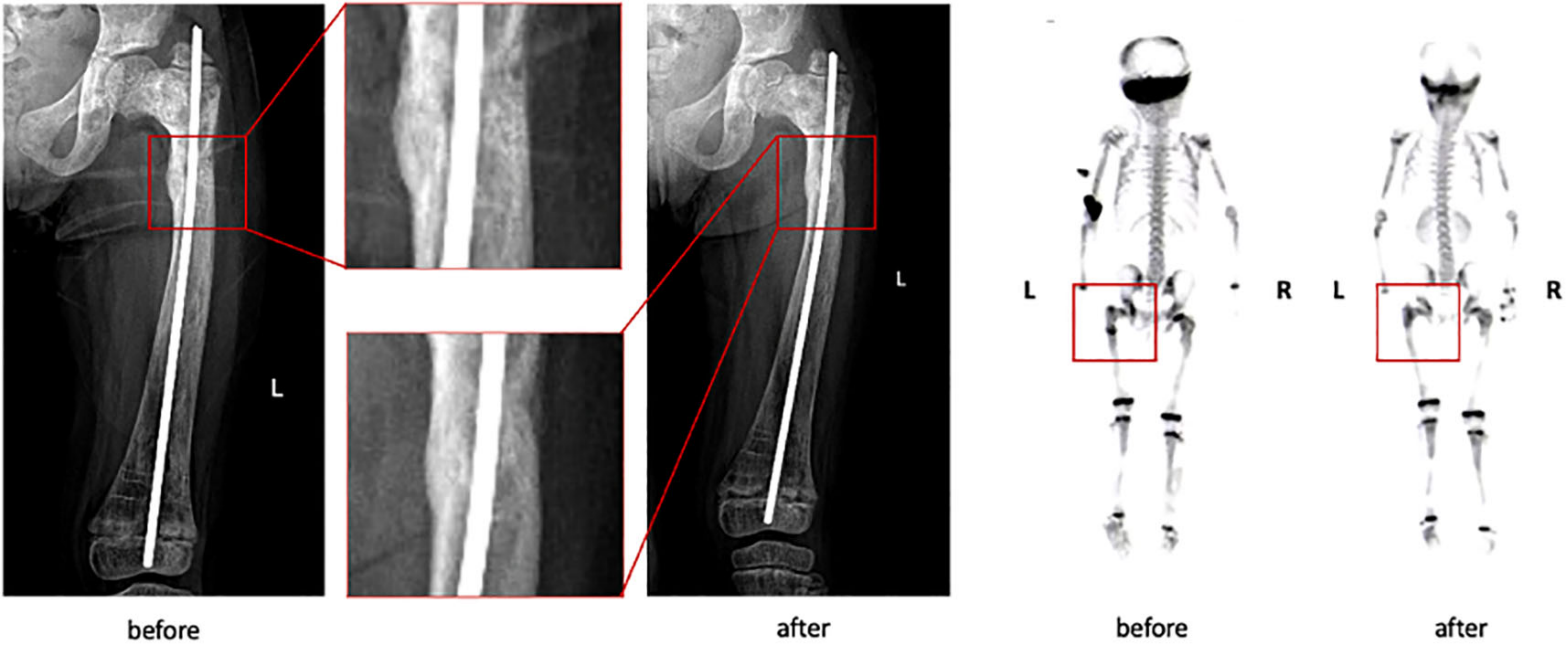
X-ray and bone scintigraphy of P2 before and after follow-up, showing reduction of left femoral and skull base lesion.
